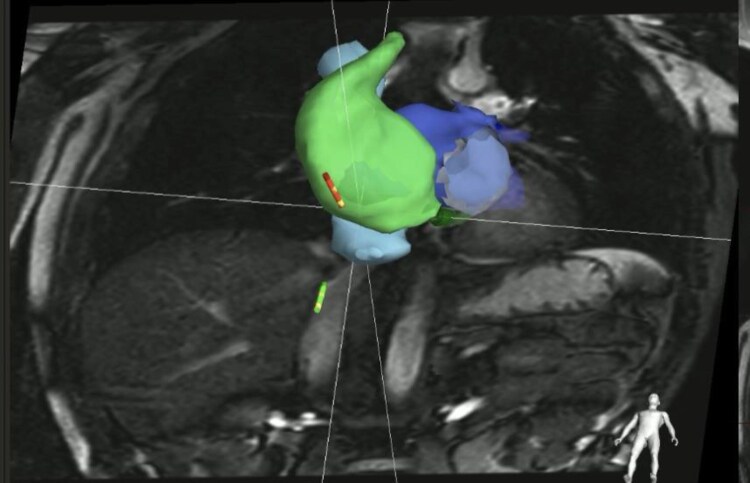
Interventional CMR-guided ablation procedure. Two catheters (green and red tip) are actively tracked and displayed on MR images and 3D reconstructed cardiac surfaces (green: right atrium, dark blue: left atrium, and light blue: superior and inferior vena cava). Courtesy of Leipzig Heart Center. CMR, cardiac magnetic resonance; MR, magnetic resonance.
